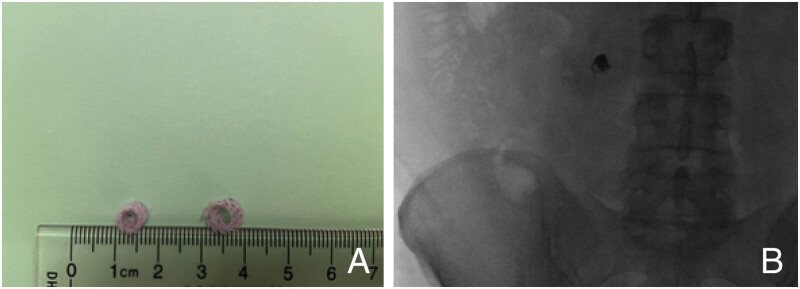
Endoscopic ultrasound (EUS)-guided vascular therapy. (A) The same coils placed transvenously by interventional radiology can also be placed via endoscopic ultrasound. (B) Here we have an endoscopically placed coil in a stomal varices, seen on post-endoscopic abdominal X-ray.
